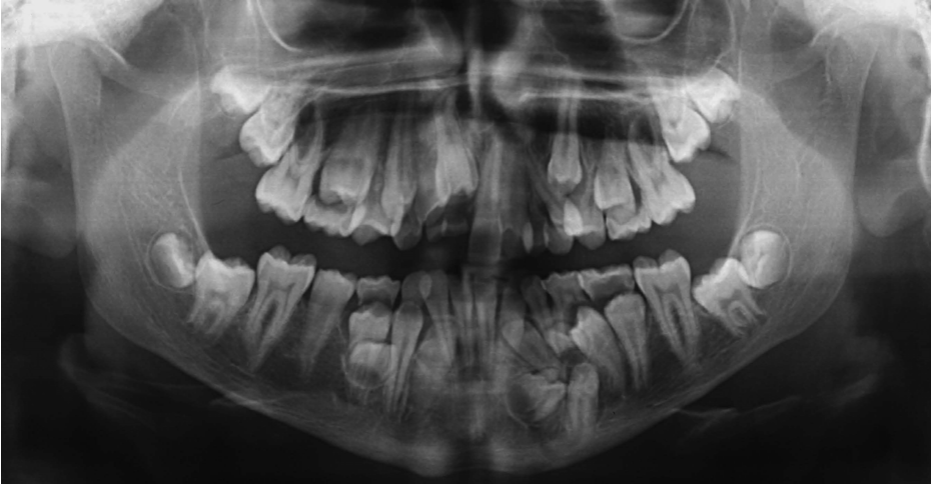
Orthopantomography at 13 years 2 months of age.
